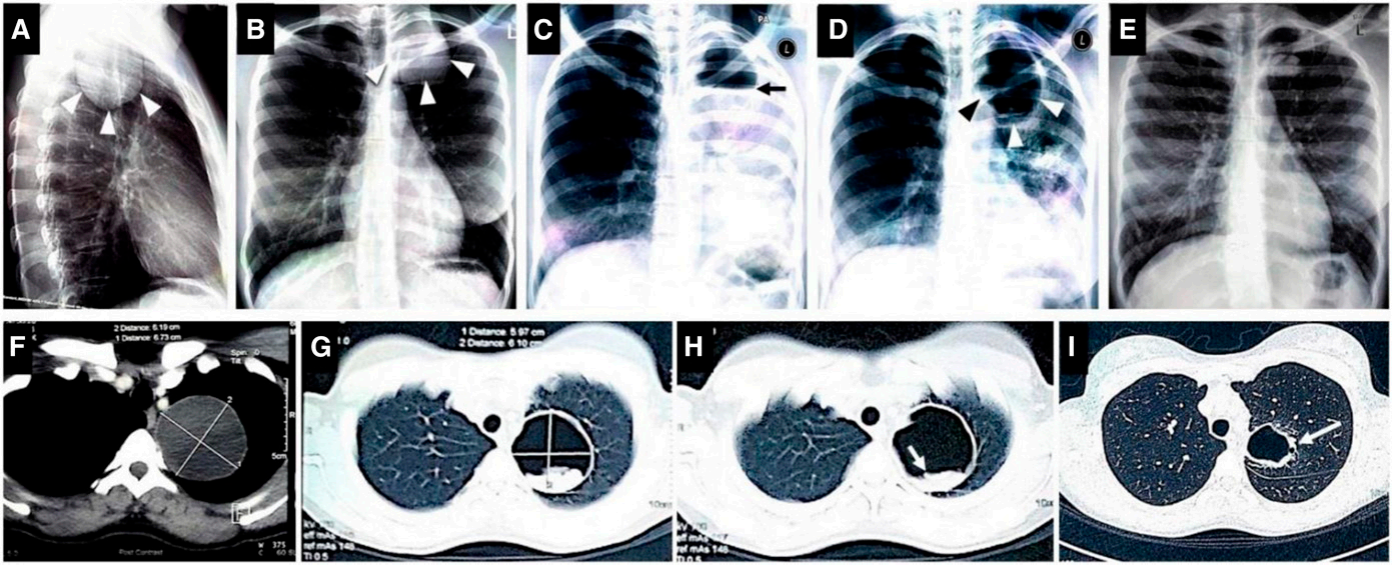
Plain radiograph (A–E) and computed tomographic (F–I) images of a 23-year-old female patient with pulmonary hydatid cyst disease. They show the large intact cyst at diagnosis (A, B, and F), left pulmonary interstitial involvement following rupture of the cyst (C), the cyst containing dependent residual tissue believed to be from the ruptured cyst wall (G and H), the cyst communicating with a bronchiole (I), and sequential evidence of recovery of pulmonary tissue (D and E). This figure appears in color at www.ajtmh.org.
